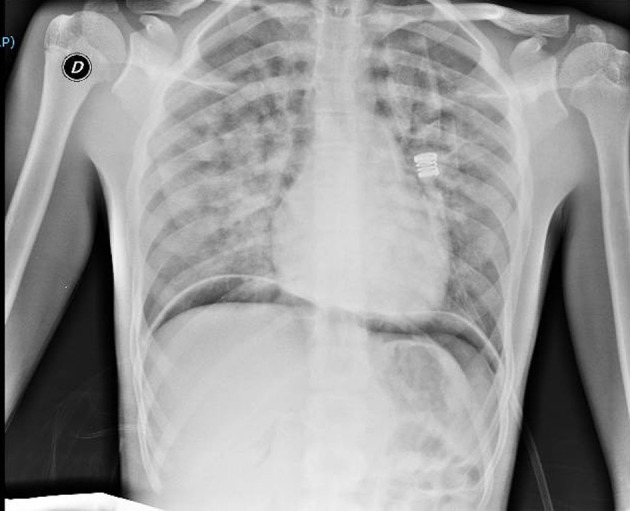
Chest x-ray at clinical onset of symptoms, consistent with diffuse alveolar hemorrhage (DAH). There can be observed infiltrative opacification pattern mainly seen in the mid zones with apical sparing suggestive for impaired pulmonary microcirculation. There can be noticed areas of subdiaphragmatic air due to laparoscopy.
